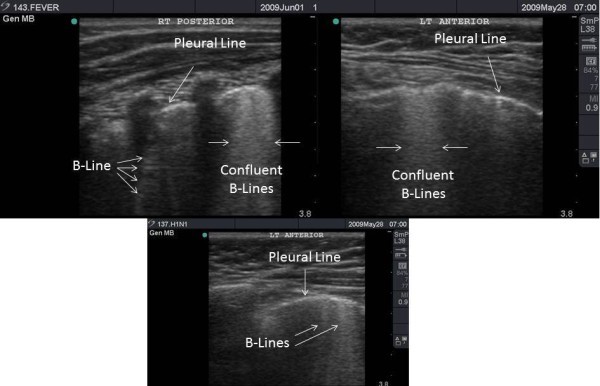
B-lines and confluent B-lines consistent with viral pneumonia lung ultrasound pattern.
